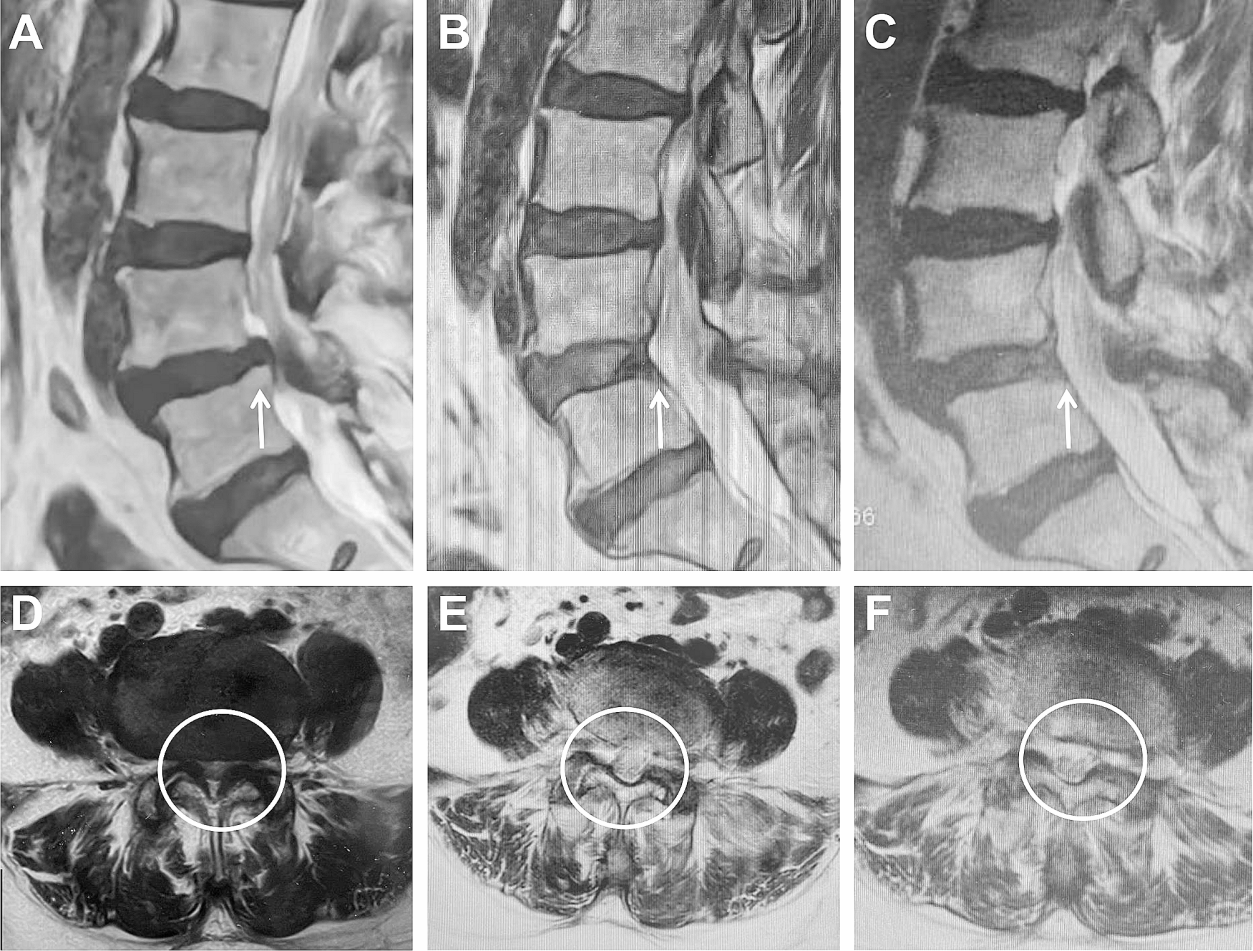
Pre- and postoperative MRI. (A) Preoperative MRI showed that DLS caused a posterosuperior protruded compression underneath the slipping vertebral body in the lumbar spinal canal, forming a stepped shape (white arrow). (D) The central spinal canal stenosis, foraminal stenosis and lateral recess stenosis (white circle). (B, E) On the third day after operation, the MRI revealed the PRSVB were fully removed (white arrow), and lateral recess, foramen and the central spinal canal were enlarged (white circle). (C, F) The MRI examination showed no evidence of significant further compression at the twelve months after surgery
